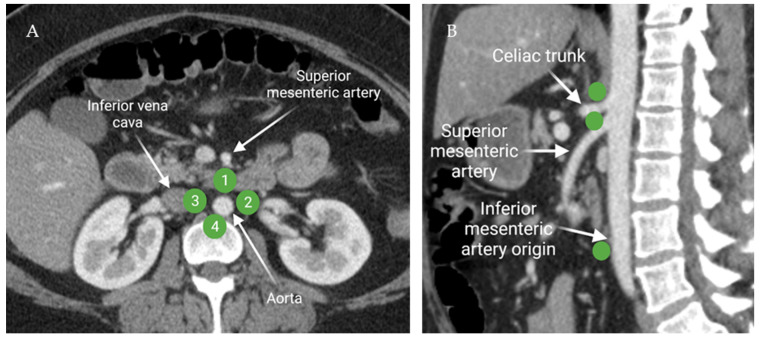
Axial contrast-enhanced CT of the abdomen (A) demonstrates the distribution of the peri-aortic lymph nodes: 1; pre-aortic, 2; left lateral aortic, 3; right lateral aortic, and 4; post-aortic. Sagittal contrast-enhanced CT of the abdomen (B) shows the distribution of the pre-aortic lymph nodes along the origins of the visceral branches of the aorta (celiac, superior mesenteric, and the inferior mesenteric arteries). “Created by BioRender.com. accessed on 10 August 2021”.
